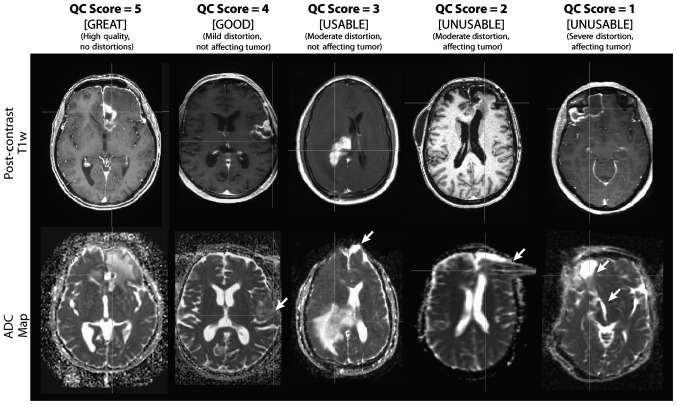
Example images for QC scores resulting from varying degrees of geometric distortion in ADC maps. QC score = 5 (great) reflects high-quality diffusion MRI data with no distortions. QC score = 4 (good) reflects mild geometric distortion that does not affect the tumor. QC score = 3 (usable) reflects moderate geometric distortion not affecting the tumor. QC score = 2 (unusable) involves images with moderate distortion that is affecting measurement of the tumor. QC score = 1 (unusable) involves severe distortion that is affecting measurement of the tumor.
